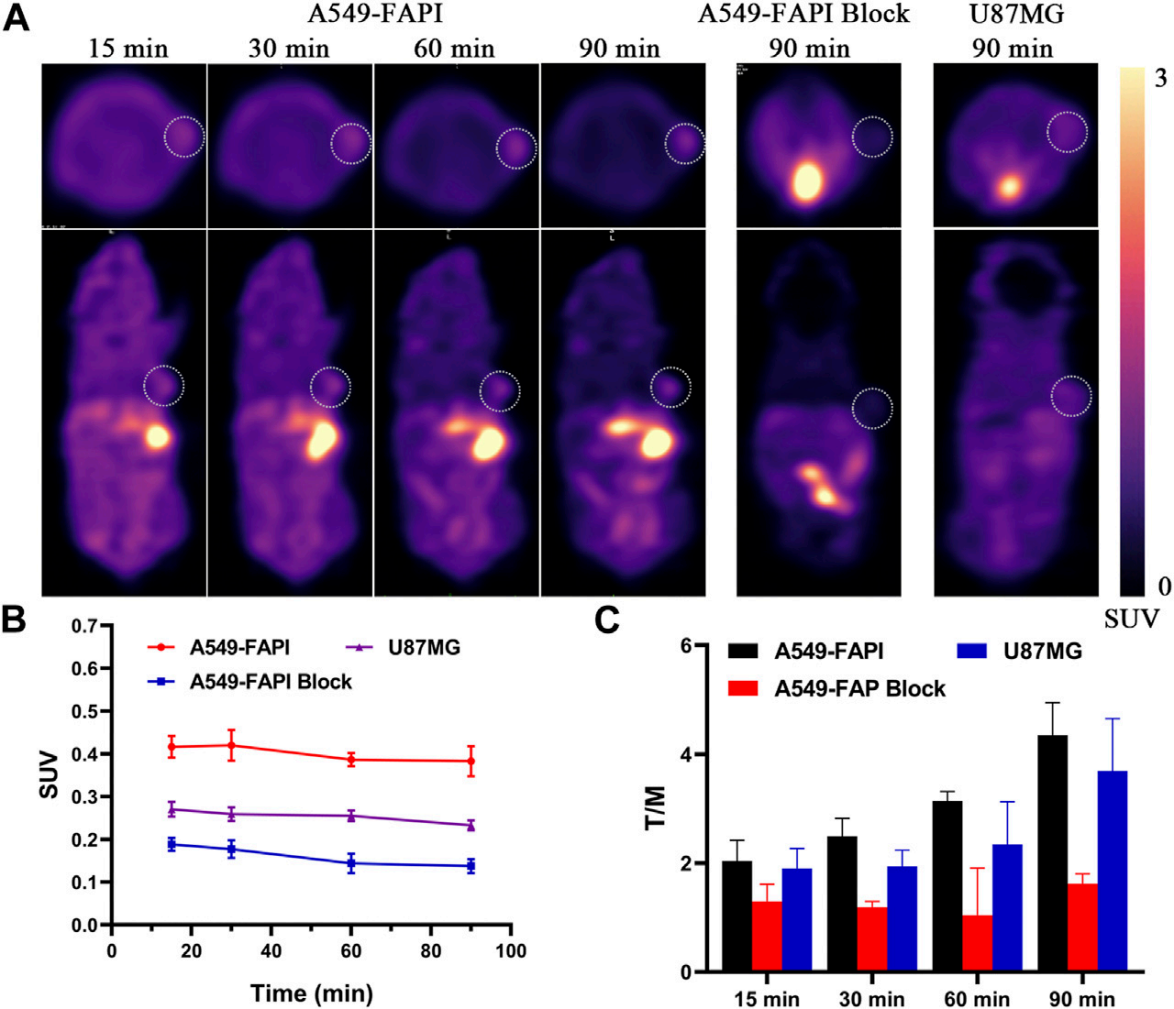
In vivo* micro-PET imaging of FAP in tumors. (A) Static micro-PET images at all time points. (B) Tumor SUVs of [^18^F]3 in all subjects; white cycles indicate the tumor lesions, and the scale bars show SUV values. (C) Tumor/muscle ratios calculated from PET images.
